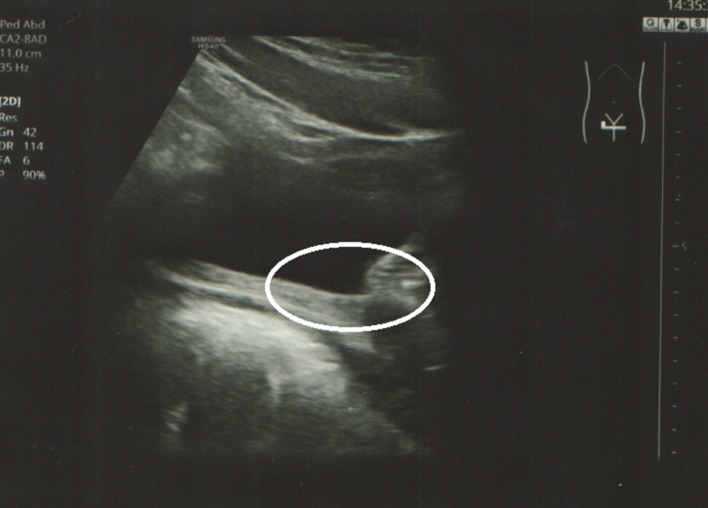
Transabdominal ultrasound: open bladder neck, sagittal view (circle).
